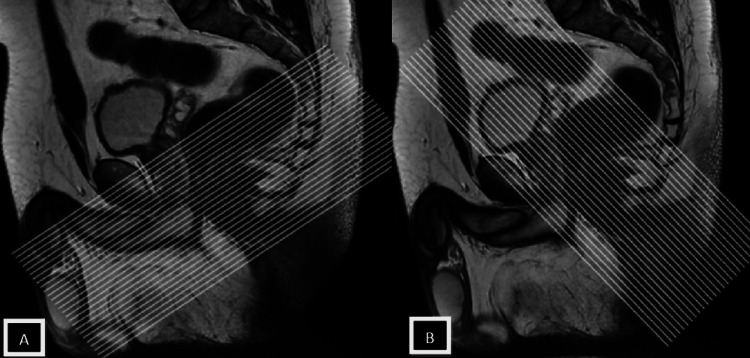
Sagittal T2-weighted midline images Sagittal T2-weighted midline images were employed in axial MR imaging of the anal canal to guarantee accurate axial alignment. For a coronal MRI, pictures were taken opposite the long hub of the butt-centric waterway, at the right point to the pivotal plane.
